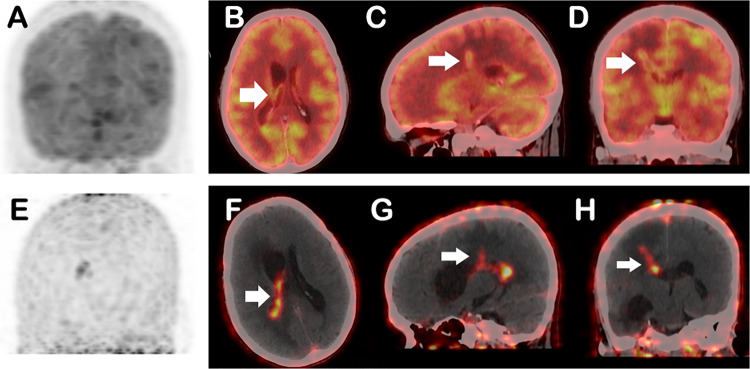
Corresponding ^18^F-FDG PET/CT (A-D) and ^68^Ga- FAPI PET/CT (E-H) images in the same patient mentioned in Fig. 4. Maximum intensity projection FDG PET image of the brain showing no definite abnormal uptake (A). Axial, sagittal and coronal ^18^F-FDG PET/CT images show ill-defined peripherally FDG avid rim enhancing lesions in the right frontal cortex with surrounding vasogenic oedema formic tract and crossing adjacent lateral ventricle with involvement of choroid plexus (arrows in B-D). Maximum intensity projection ^68^Ga- FAPI PET image of the brain shows heterogenous tracer uptake in the right cerebral cortex. Axial, sagittal and coronal ^68^Ga- FAPI PET/CT images showing FAP expressing lesions in the right frontal cortex with surrounding vasogenic oedema forming tract and crossing adjacent lateral ventricle and involving choroid plexus (arrows in F-H)
